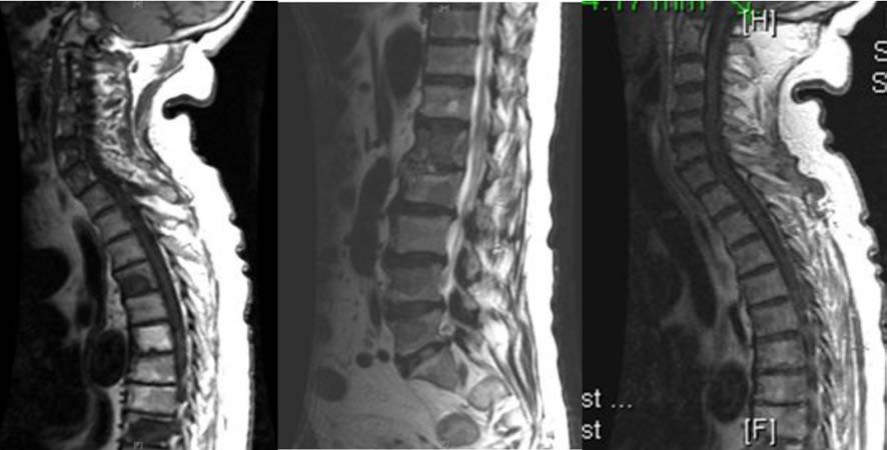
MRI of the spine showing multi-level osseous metastatic infiltration throughout the spinal axis particularly involving the lower thoracic and lumbar regions.
